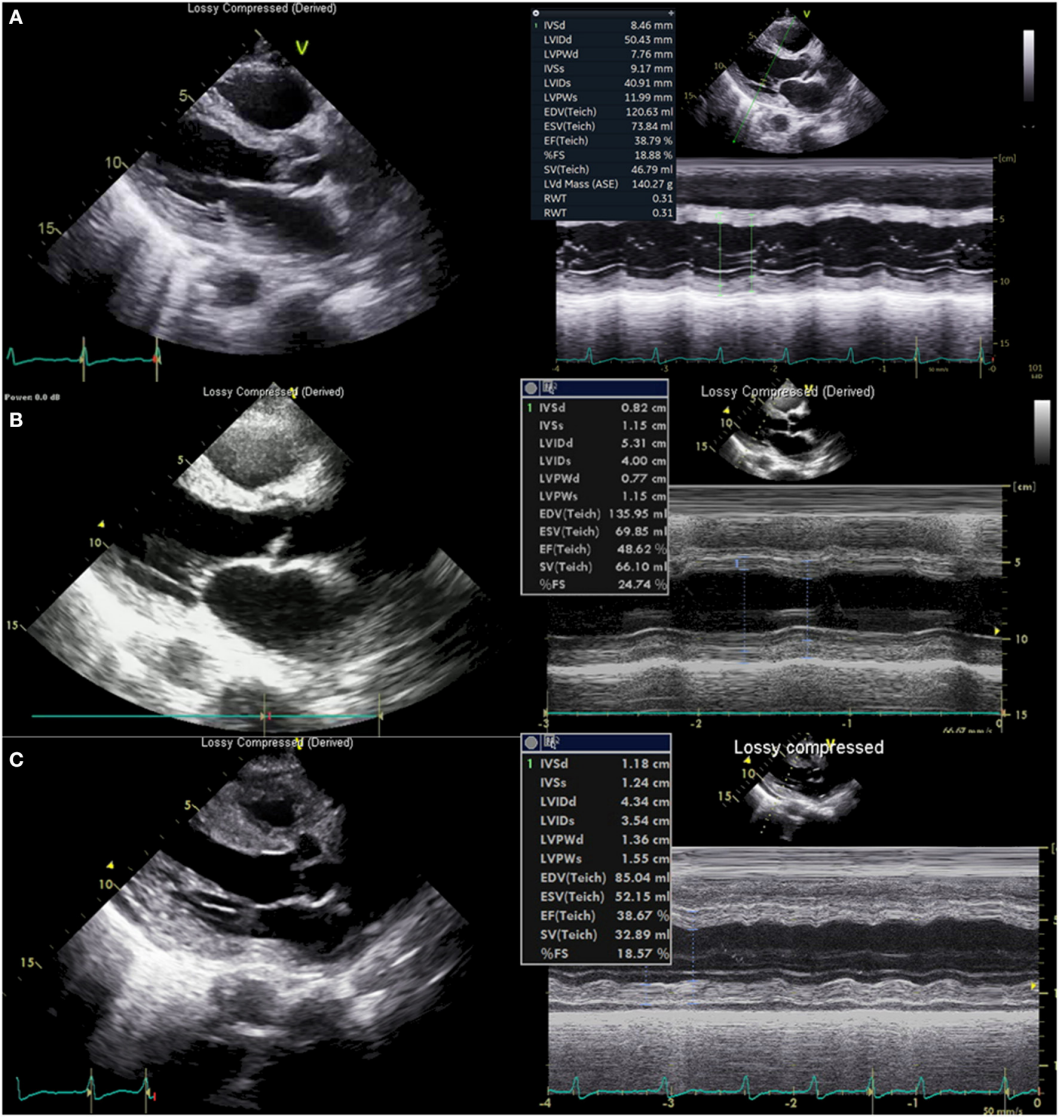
Echocardiography. (A) Echocardiography at presentation showed normal left ventricular (LV) wall thickness and chamber size. (B) Echocardiography 6 h before cardiac arrest showed normal LV wall thickness. (C) Echocardiography just after extracorporeal CPR showed thickened LV (13.6 mm, posterior wall, 11.8 mm septum) and right ventricle wall.
